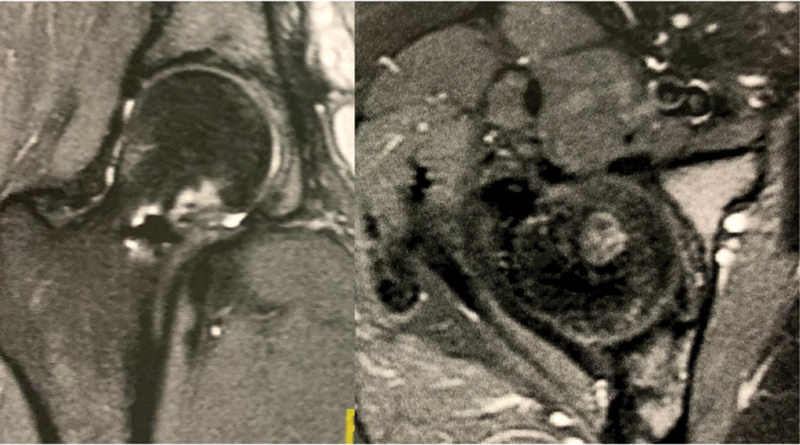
MRI 1 year after surgery, demonstrating scar tissue with bone remodeling.
